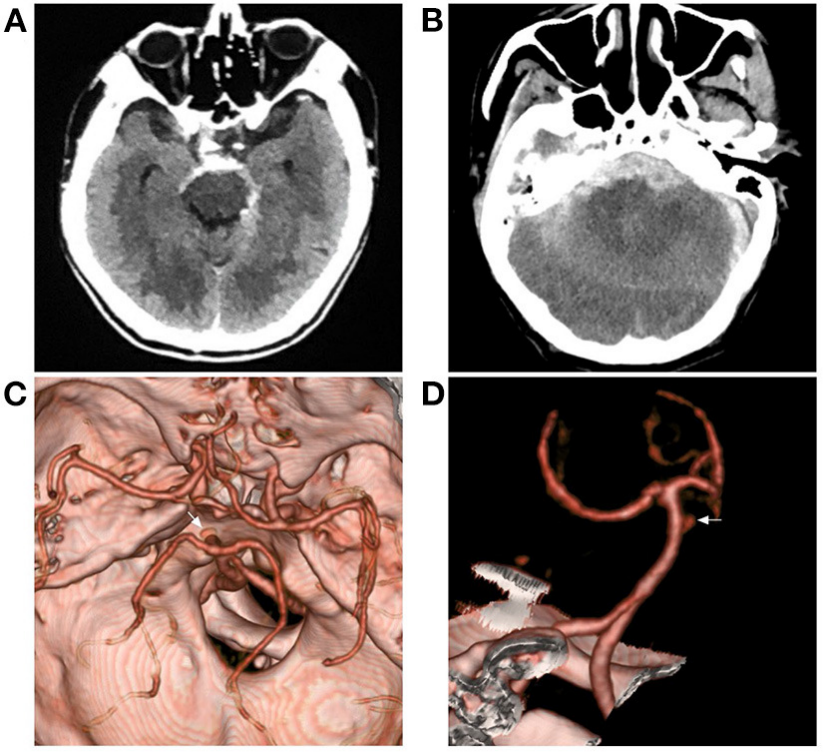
Aneurysmal SAH with perimesencephalic bleeding pattern. (A) Immediate CT of the onset showing SAH with typical perimesencephalic bleeding pattern. (B) Repeated CT 6 h after the onset showing a second SAH with the thick hemorrhage in front of the brainstem. (C,D) CTA showing an aneurysm of the basilar artery (arrows). CT, computed tomography; CTA, computed tomography angiography; SAH, subarachnoid hemorrhage.
